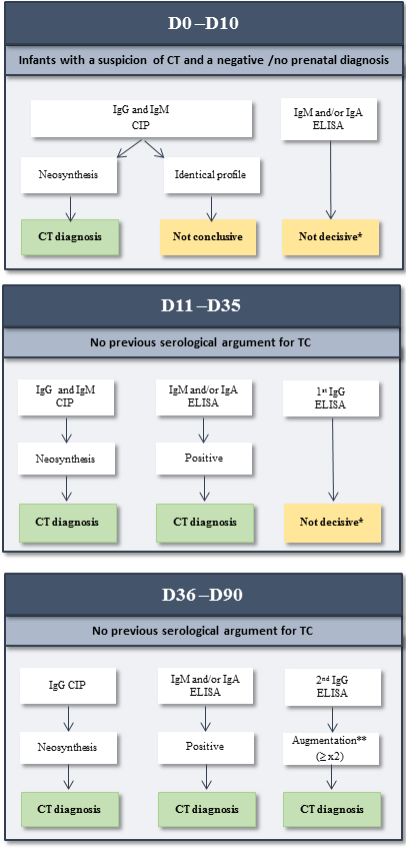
Recommandations from National Reference Center to CT serological diagnosis on an infant with negative or no prenatal diagnosis. *Risk of contamination by maternal immunoglobulins. IgG must be measured in parallel on successive sera with the same technique.
